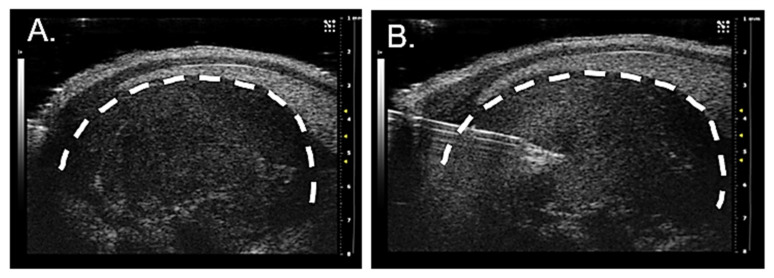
Representative ultrasound images of (A) transverse view of intraperitoneal tumor in lateral lower right quadrant of the abdomen showing skin line and fat under the skin. (B) Placement of the needle within the tumor for injection. Dotted line highlights tumor.
